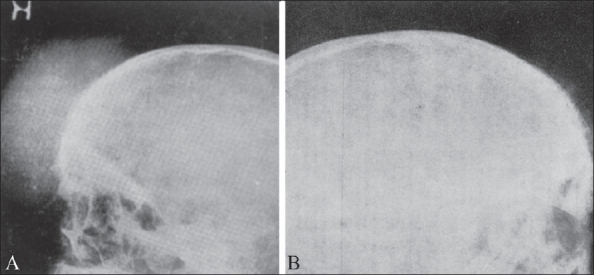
Ewing's sarcoma of the frontal bone. The pre-irradiation plain radiograph (A) of the skull shows condensation of bone in the frontal region with a large soft tissue swelling. The post-irradiation radiograph shows virtually complete regression of the soft tissue
